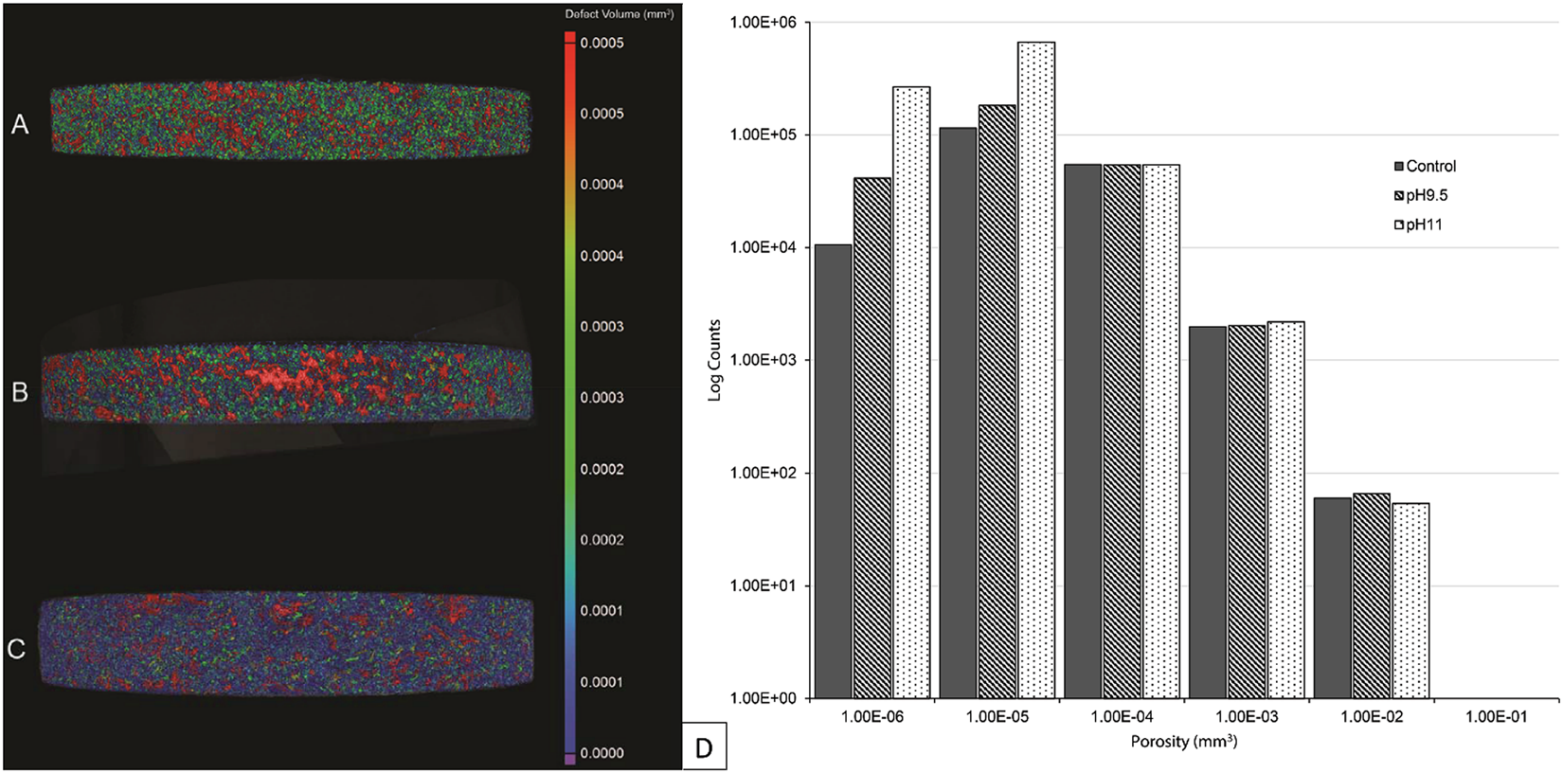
CT scanning of ^13^C doped simulants. (Left) The simulant surface prior to insertion to microcosms can be seen in (A), the pH 9.5 (B) and pH 11.0 (C) microcosms associated surfaces are also shown. The associated defect volumes and Log counts associated with those volumes of each of the surfaces is also shown (D).
